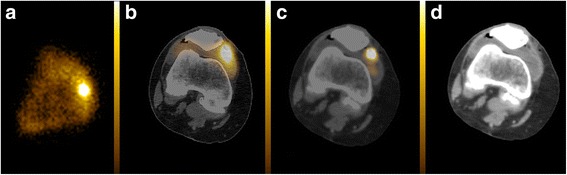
Anterior bremsstrahlung planar (a), axial fused SPECT/CT (b), axial fused PET/CT (c) and co-registered CT (d) images of the right knee demonstrating a predominantly diffuse but also focal (non-diffuse) planar imaging Y-90 pattern with the focal component of the Y-90 activity located extra-articularly on fused PET/CT (superficial to the deepest capsular layer but deep to the medial patellar retinaculum). SPECT/CT was unable to definitively localise the focal component to the intra- or extra-articular space
